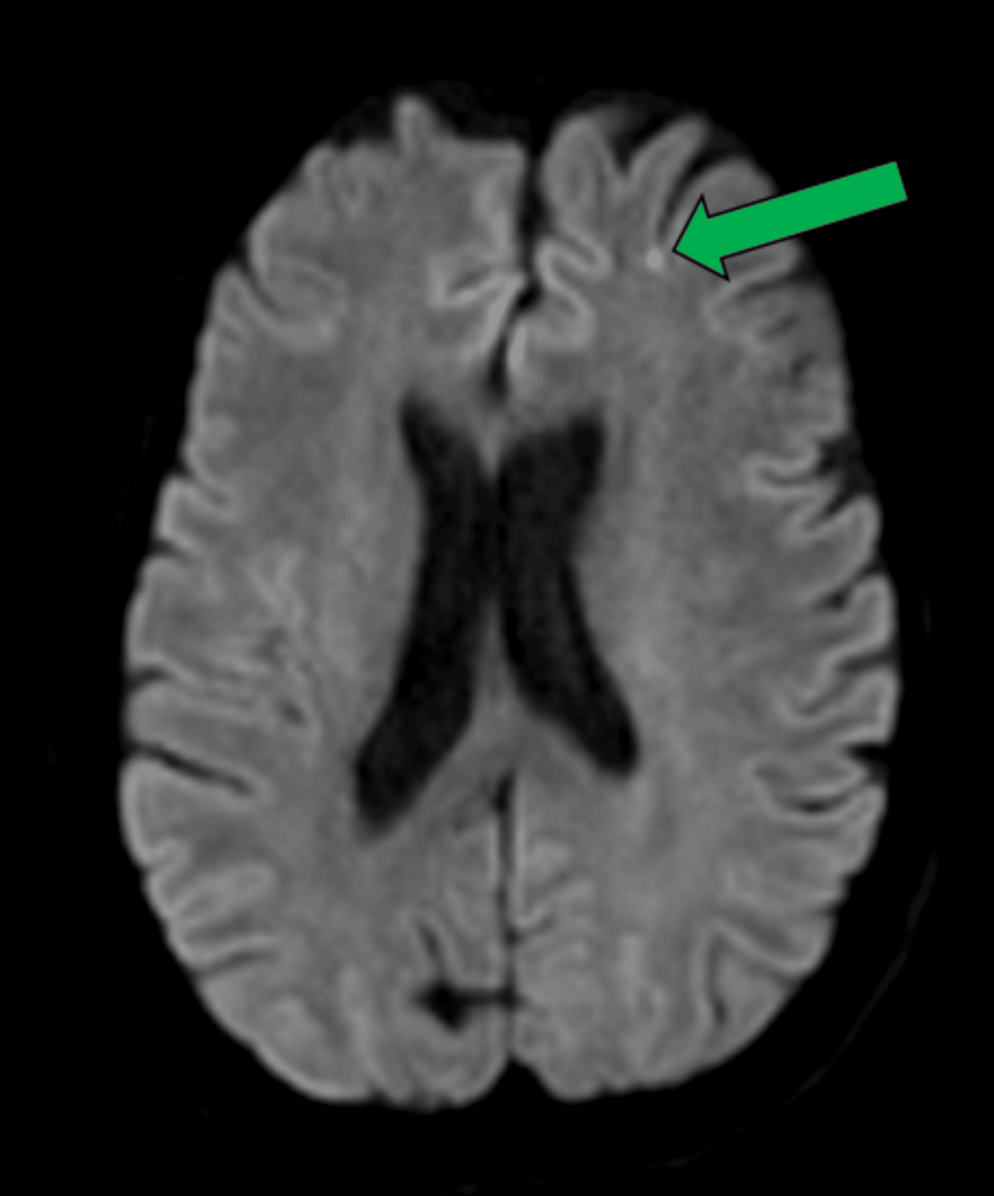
MRI axial DWI and ADC of the brain at the level of the lateral ventricles demonstrate a small focus of hyperintense DWI in the left frontal lobe (green arrow). These findings suggest a small subacute infarct. MRI: magnetic resonance imaging; DWI: diffusion-weighted imaging; ADC: apparent diffusion coefficient
